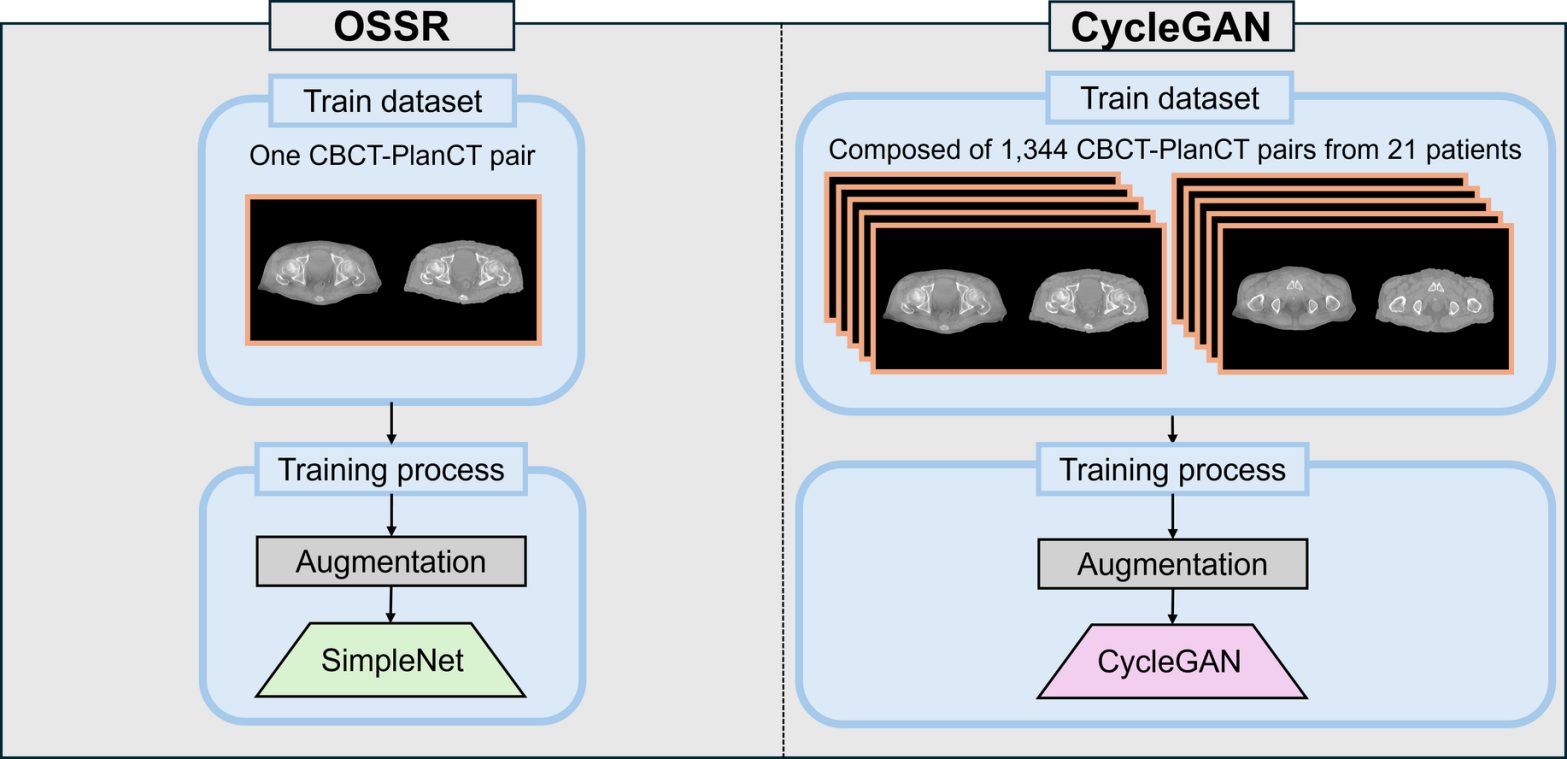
Comparison of the training dataset used for the training of OSSR and the conventional learning method CycleGAN. OSSR trains the model using only a single CBCT–PlanCT pair, whereas CycleGAN trains the model with 1344 CBCT–PlanCT pairs from 21 patients
